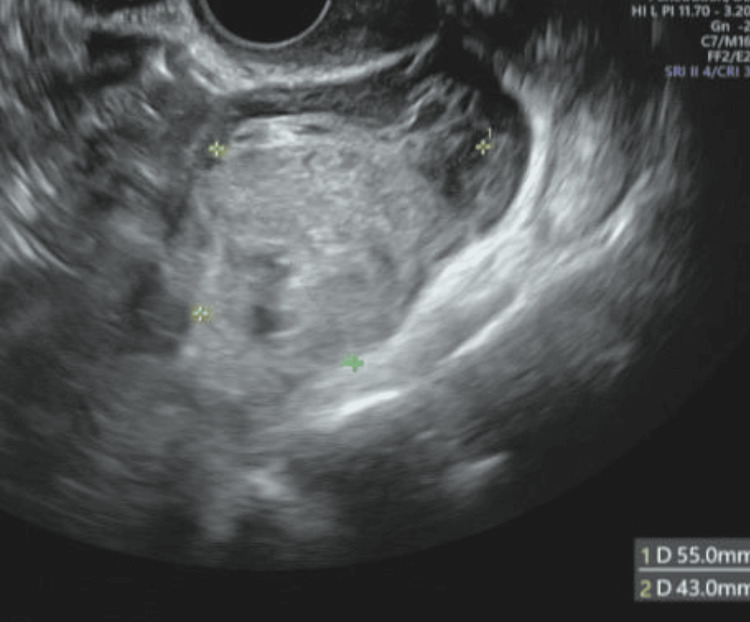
Transvaginal ultrasound. A hematoma measuring 55 mm x 43 mm around the left adnexa.
